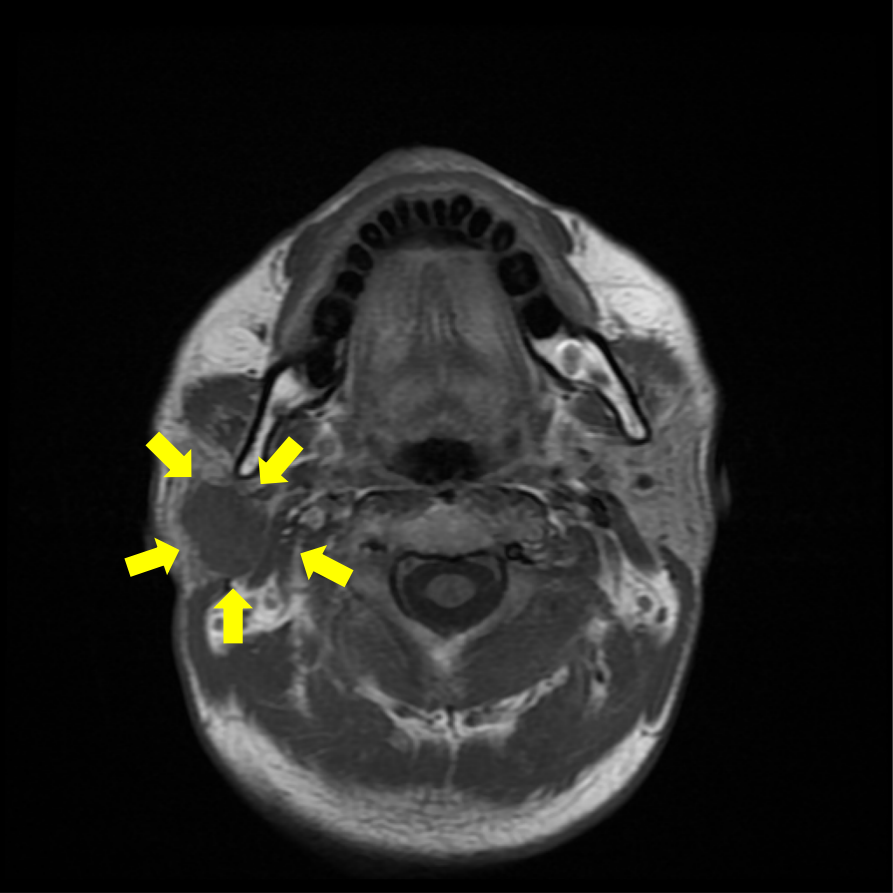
T1-weighted magnetic resonance imaging (MRI) showing a low-enhanced, well-defined mass in the right parotid gland (arrow)
